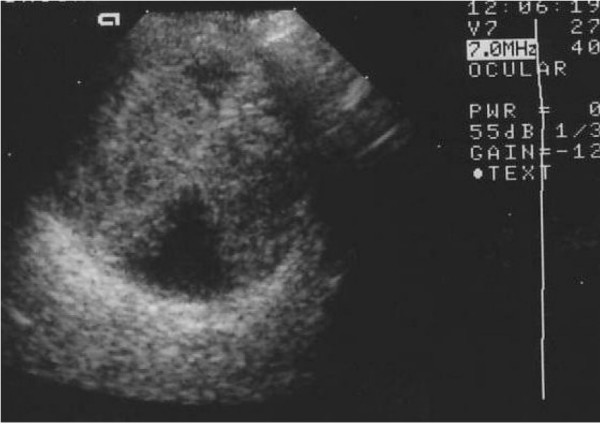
Dense echogenic shadow due to suprachoroidal haemorrhage on ultrasonography of the right eye (B scan,10 Hz).
